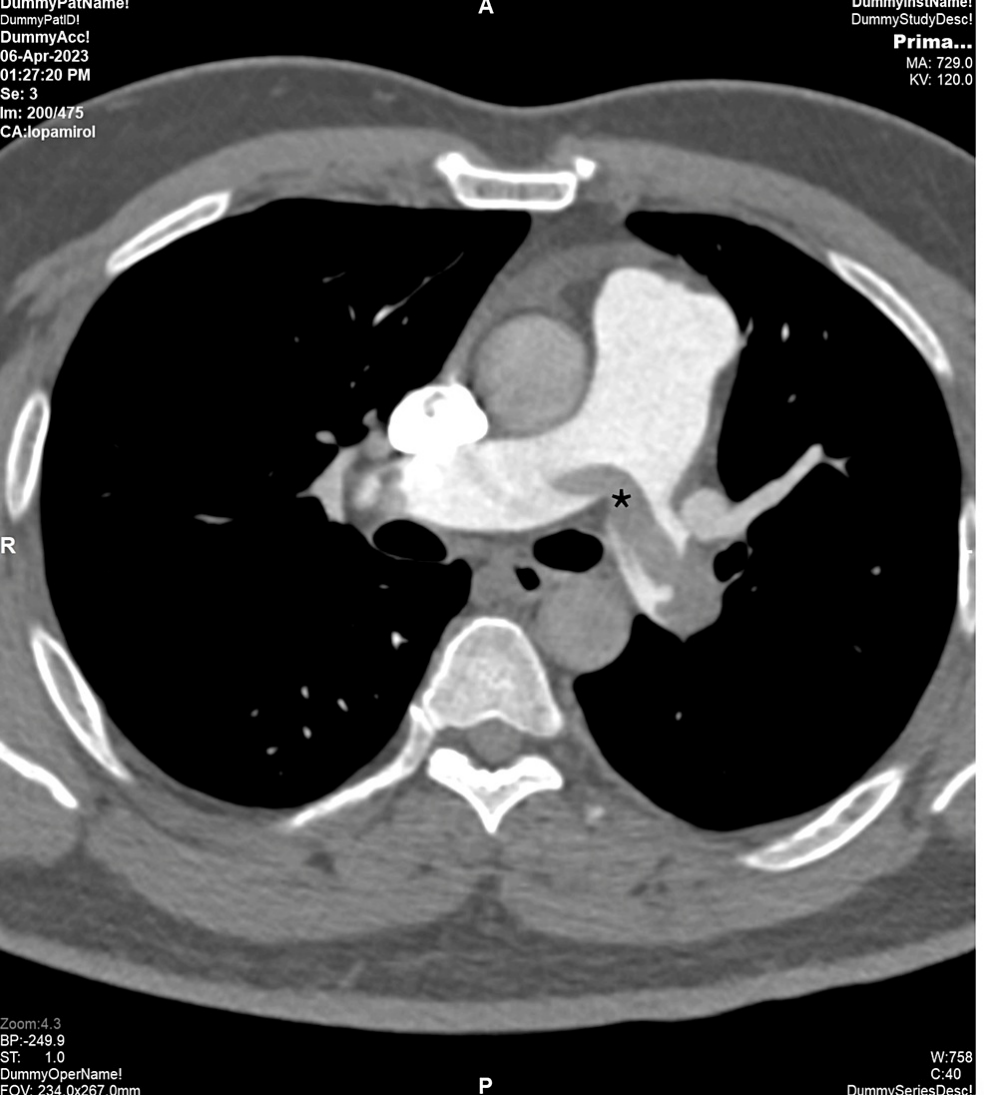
Axial CPTA at the level of the main pulmonary artery bifurcation shadowing a large filling defect (labeled with an asterisk) straddling the main pulmonary artery extending to the right and left pulmonary arteries consistent with saddle embolism Note the dilation of the pulmonary artery relative to the corresponding ascending aorta. CPTA: CT pulmonary angiography
